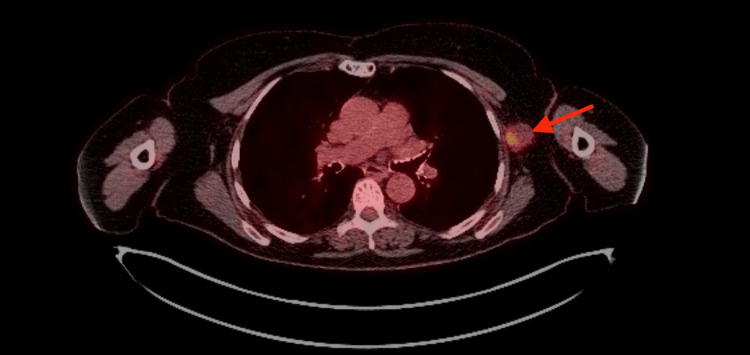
PET scan reveals axillary uptake (arrow) with maximum SUV (standardized uptake value) of 6.3.
